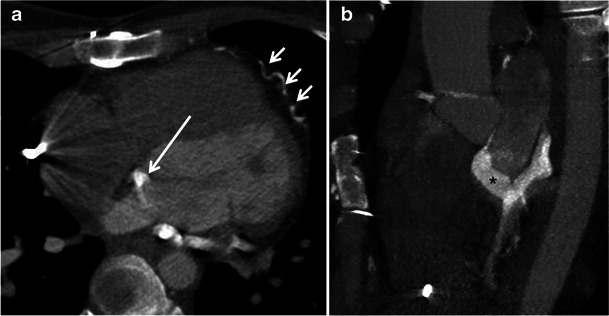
A 26-year-old female with Ebstein anomaly, CS ostial atresia and a persistent LSVC draining into the left atrium via the CS. a, b Multiplanar reformat CT images demonstrate communication between the CS and the left atrium (a, *long arrow*) via an anomalous vein (b, *asterisk*) without connection to the right atrium. Coronary venous drainage occurs through a network of collateral veins feeding directly into the right atrium (a, *short arrows*)
